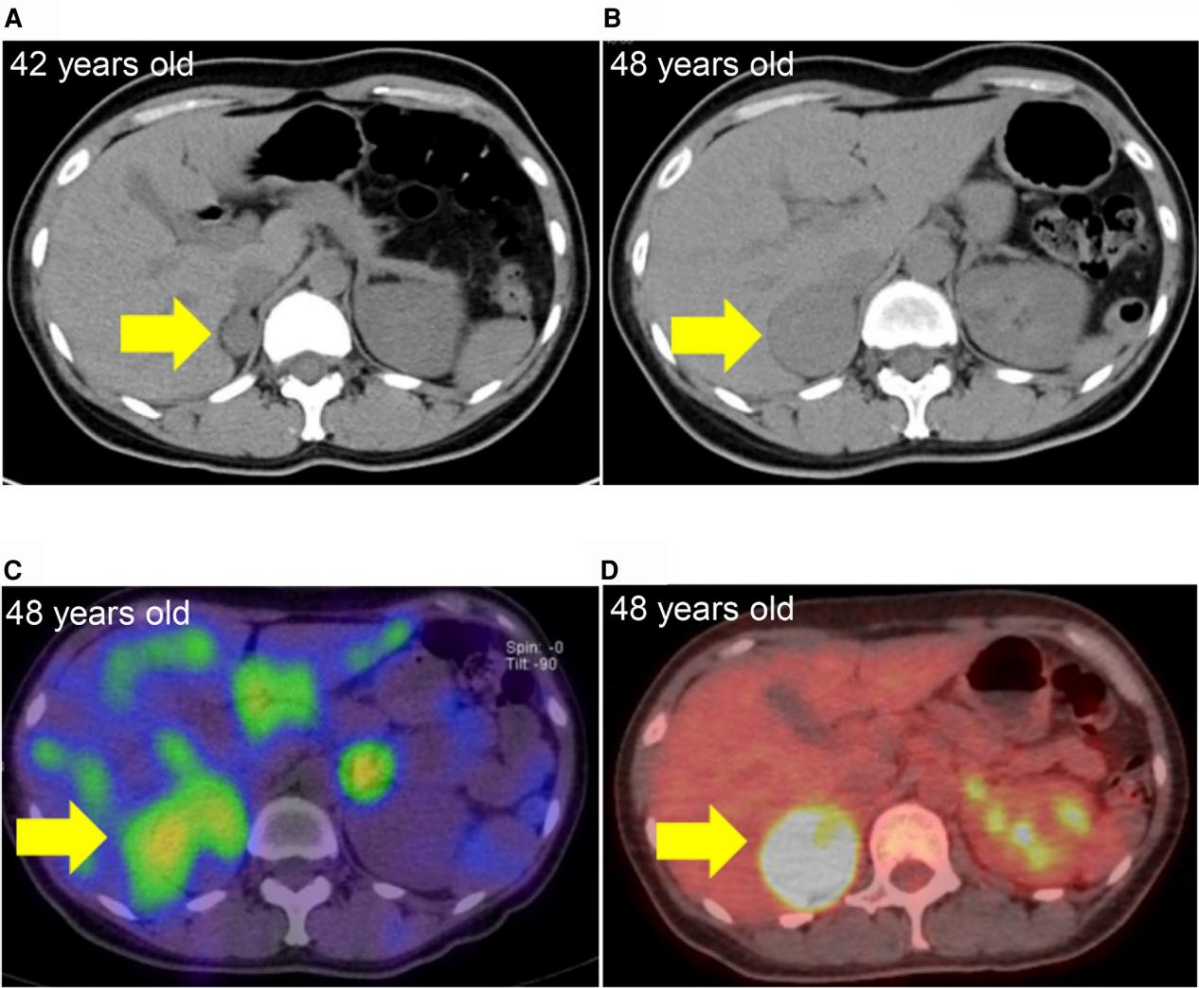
Image findings of the right adrenal gland. A, Computed tomography (CT) image at age 42 years. The arrow indicates a 22-mm tumor. The CT value of the tumor was 36 HU. B, CT image at age 48 years. The arrow indicates a 42-mm tumor. The CT value of the tumor was 43 HU (C) ^123^I-MIBG scintigraphy at age 48 years. The arrow indicates a tumor in the right adrenal gland. D, FDG-PET at age 48 years. The arrow indicates a tumor in the right adrenal gland.
